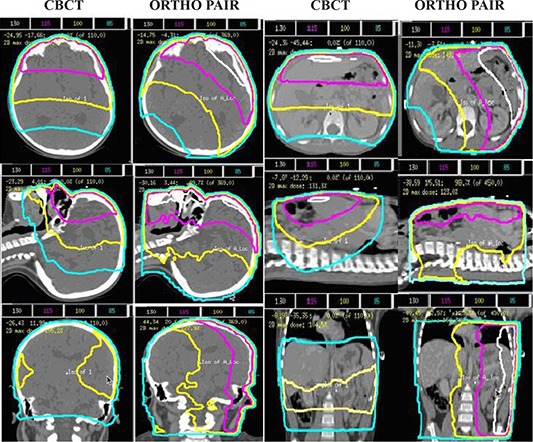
The dose distributions, normalized to isocenter, on transverse, coronal and sagittal planes along the isocenter of a cranial and a body patient for an IBL CBCT and ortho‐pair plan.
